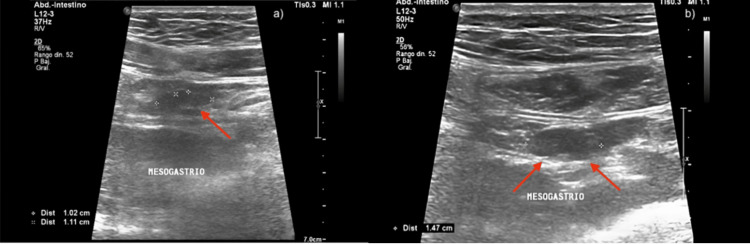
Abdominal ultrasound. (a) Several lymph nodes with swollen appearance are observed. (b) A swollen lymph node measuring 14 mm, suggestive of mesenteric adenitis, and a small amount of free fluid in the right iliac fossa are noted.
